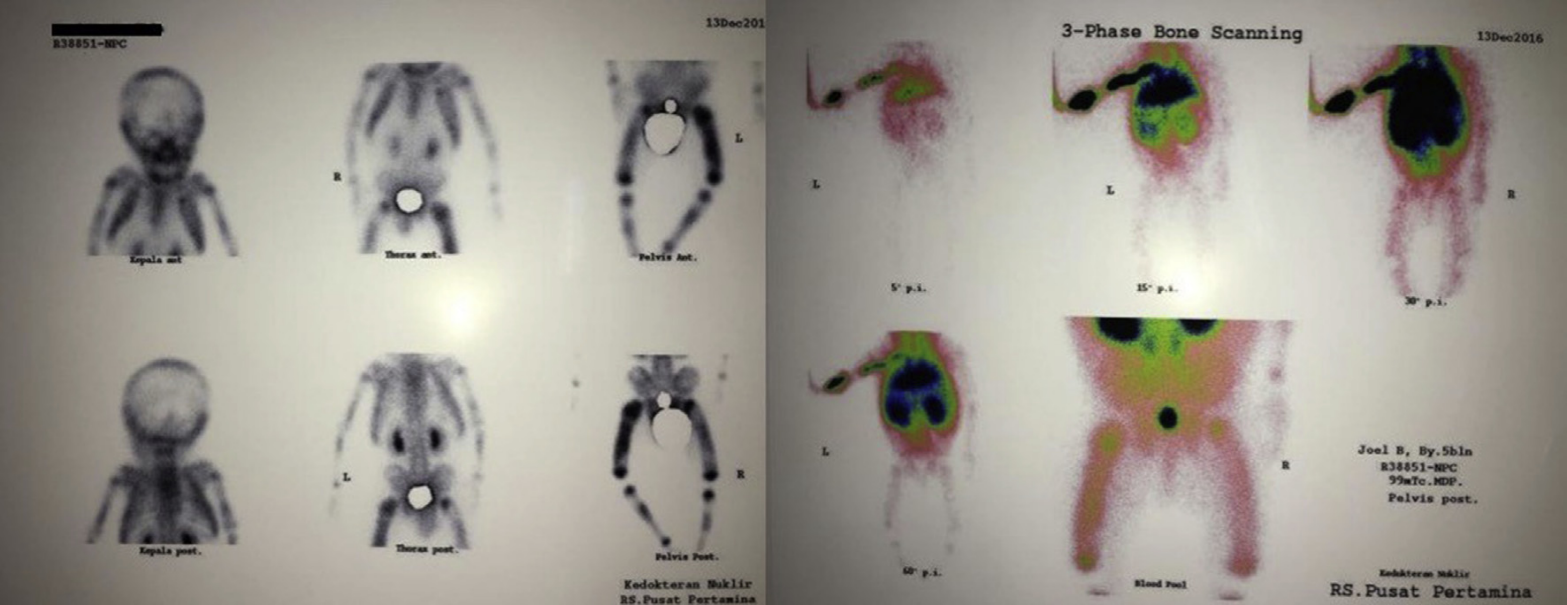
Bone scan examination supported the first MRI result.
